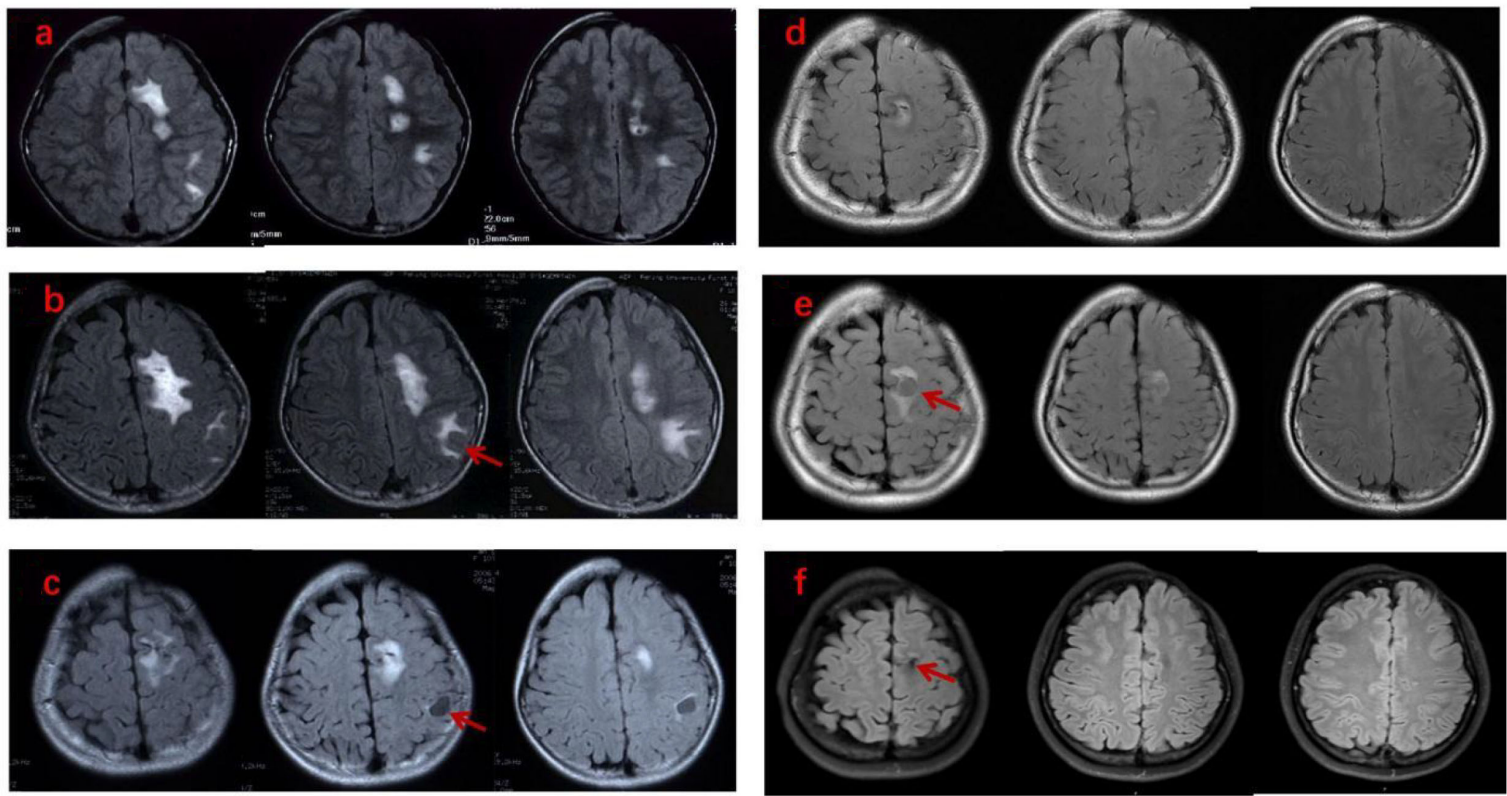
Evolution of neuroimaging findings in a patient with LS ECDS (Case 1). Axial T2-weighted fluid-attenuated inversion recovery (FLAIR) sequences showed multifocal hyperintensities in the left frontal and parietal lobes, which were ipsilateral to the facial skin lesions. Cyst-like lesions(arrowhead)appeared, resolved, and then reappeared in different locations, ultimately regressing during the 15-year follow-up period. (a, 2 years; b, 3 years; c, 4 years; d 8 years; e, 9 years; f, 15 years of follow-up).
